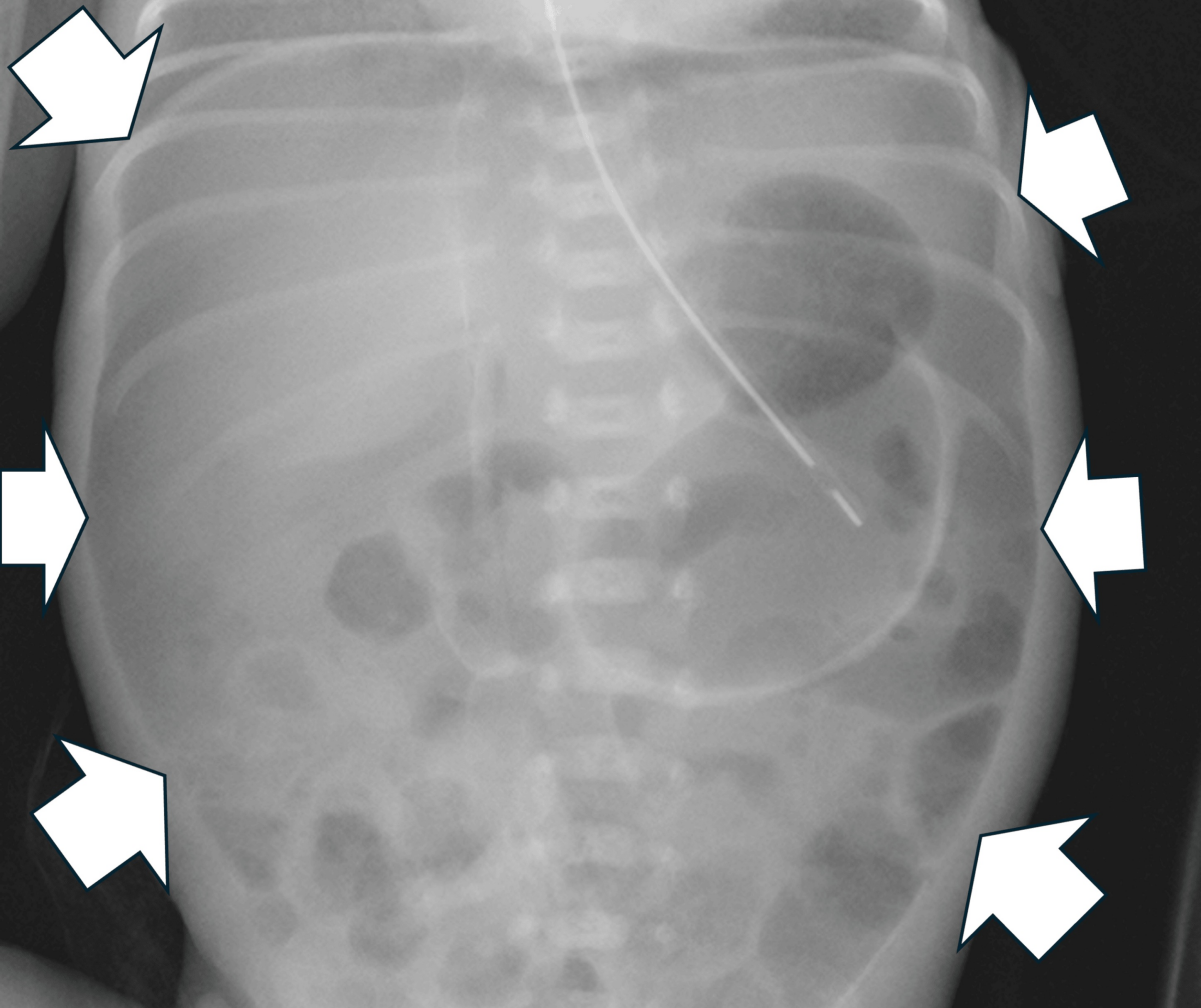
Abdominal radiograph finding in the supine position on day of life eight. Figure shows the air dome sign on a supine (white arrow).
